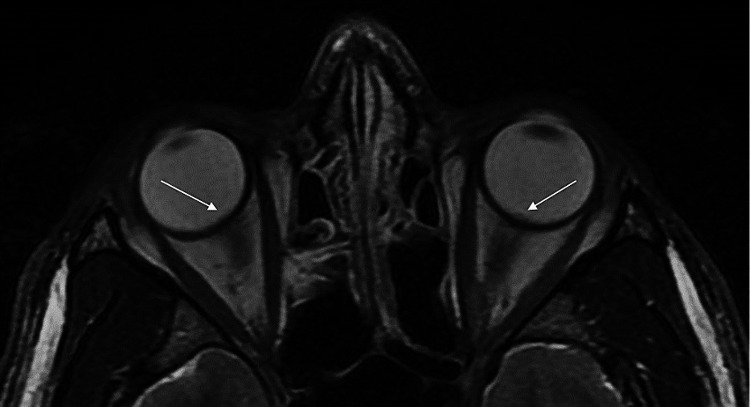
MRI T2-weighted sequencing of the bilateral orbits MRI image showing protrusion of bilateral optic nerves into the globes consistent with papilledema (arrows)
